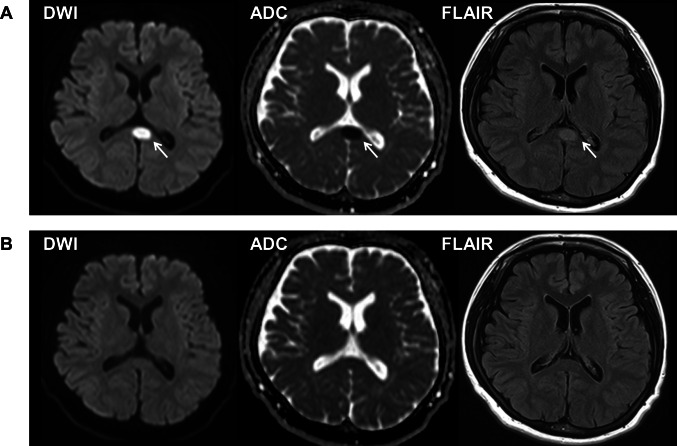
Magnetic resonance images of the brain of Patient 2 on Day 17 (A) and on Day 29 (B) after COVID-19 vaccination. A Diffusion-weighted image (DWI) (left) shows restricted diffusion in the splenium with low apparent diffusion coefficient (ADC) values (middle), and fluid-attenuated inversion recovery image (FLAIR) (right) shows a high signal intensity lesion at the midline of the splenium of the corpus callosum (arrows). B The lesion disappeared after intravenous high-dose methylprednisolone therapy
